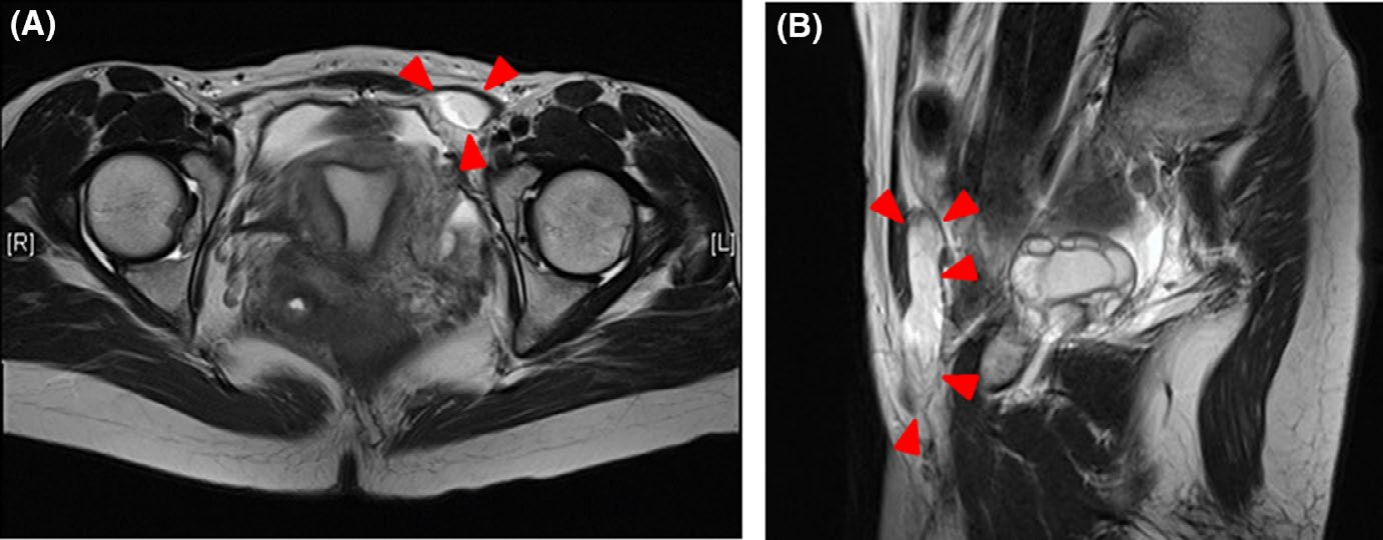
Magnetic resonance imaging findings. (A) Transverse T2‐weighted and (B) sagittal T2‐weighted images demonstrate an elongated tubular cystic lesion of 10 × 50 mm in size found in the left inguinal region (red arrowhead)
